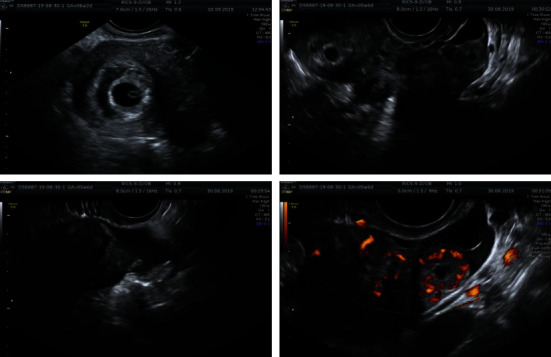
Simultaneous sonographic appearance of intrauterine and tubal ectopic pregnancy and free fluid in the Douglas pouch.
